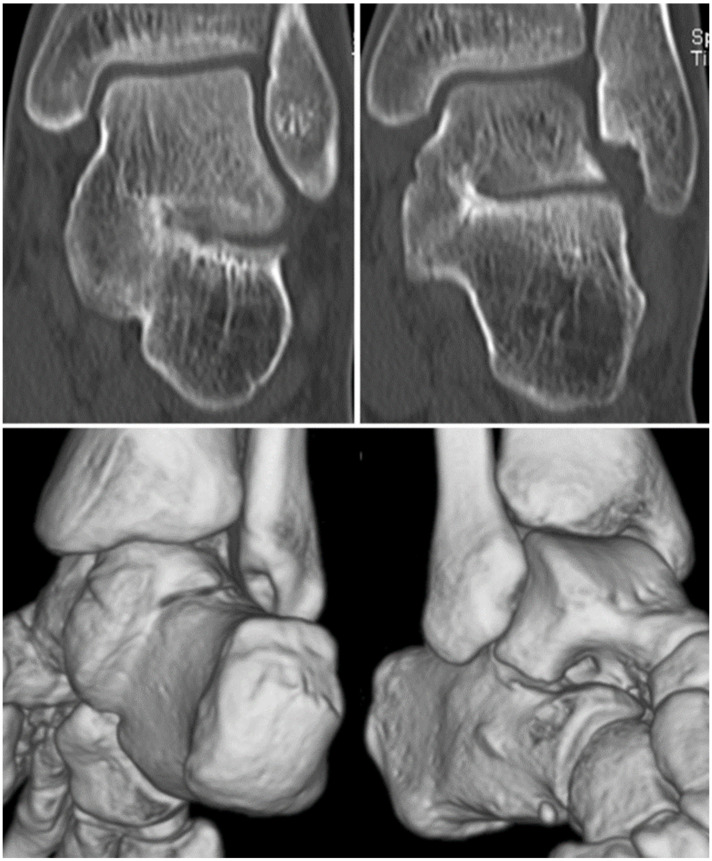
Talocalcaneal coalition, as viewed from two coronal CT sections (upper images) and three-dimensional (3D) reconstruction (lower image). Abnormal bony coalition is noted at the medial aspect of the posterior facet of the subtalar joint [10] (Borrowed using Creative Commons licensing).
